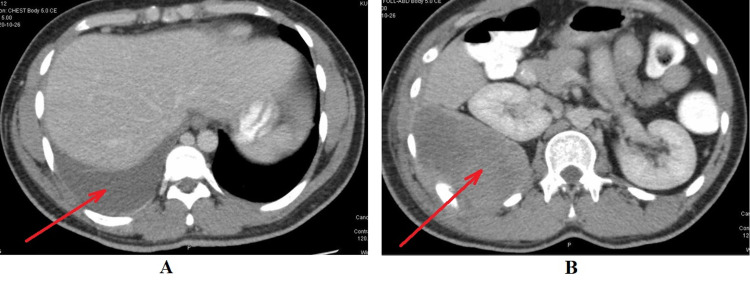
CT scan of the chest, abdomen, and pelvis. A: Mild to moderate right-sided pleural effusion (red arrow). B: Solid heterogeneous soft tissue density lesion (red arrow) along the right lower posterolateral chest wall.
